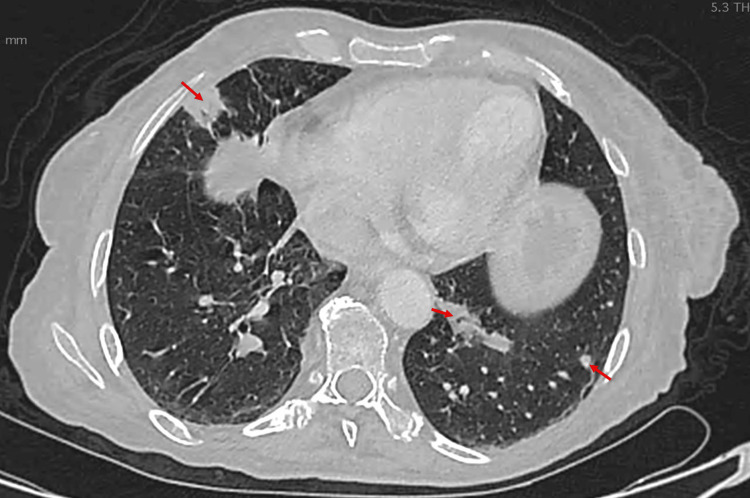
Case 1: Axial chest CT demonstrating multiple bilateral pulmonary nodules (arrows) with peribronchovascular and peripheral distribution.
